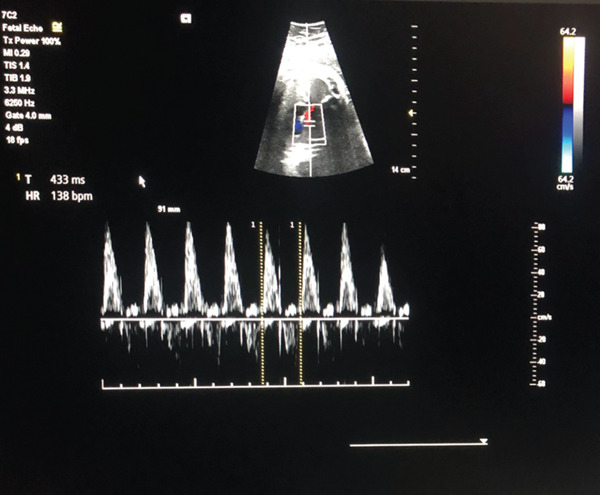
Pulse wave Doppler fetal echocardiography obtained from a five‐chamber view. The heart rate was 138 bpm after 48 h of therapy.
